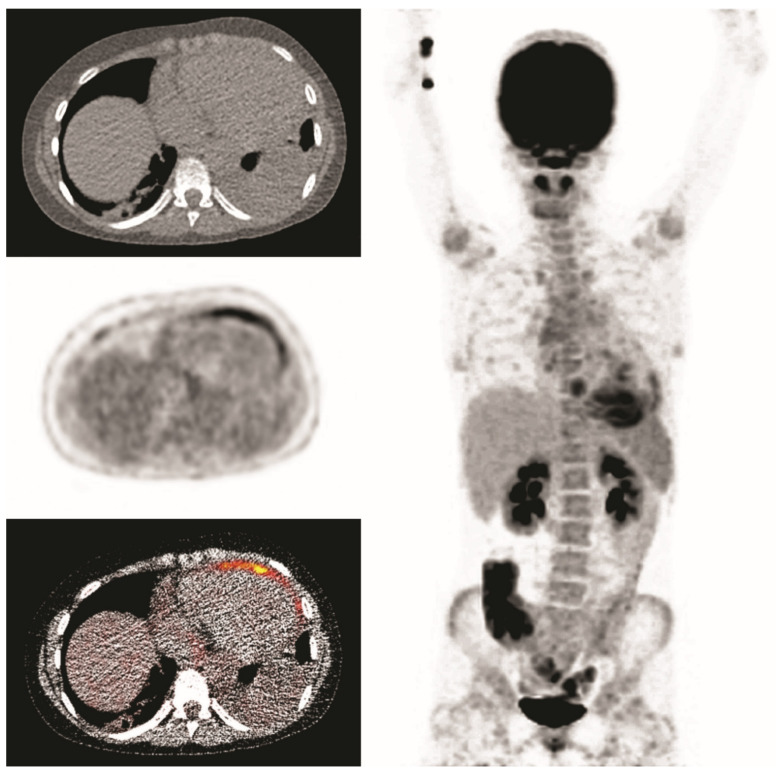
Clinical example 1. Pericardial FDG-uptake is present, most pronounced around the apex and anterior wall.
